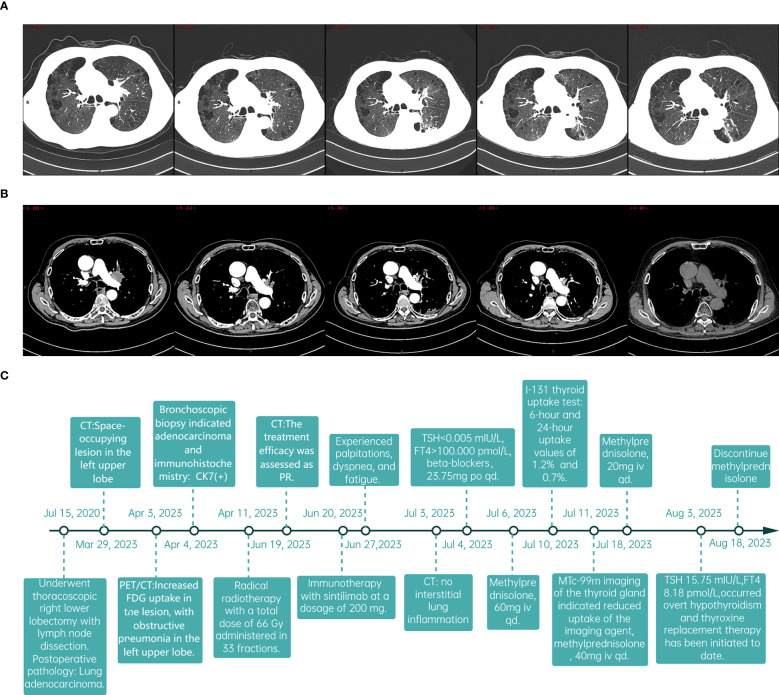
The follow-up data of patients and the timeline. (A, B) Sequentially from left to right, the dates indicate the patient’s CT scans: March 29, 2023; June 19, 2023; September 13, 2023; January 25, 2024; and September 3, 2024. (C) The timeline with relevant data from the episode of care.
